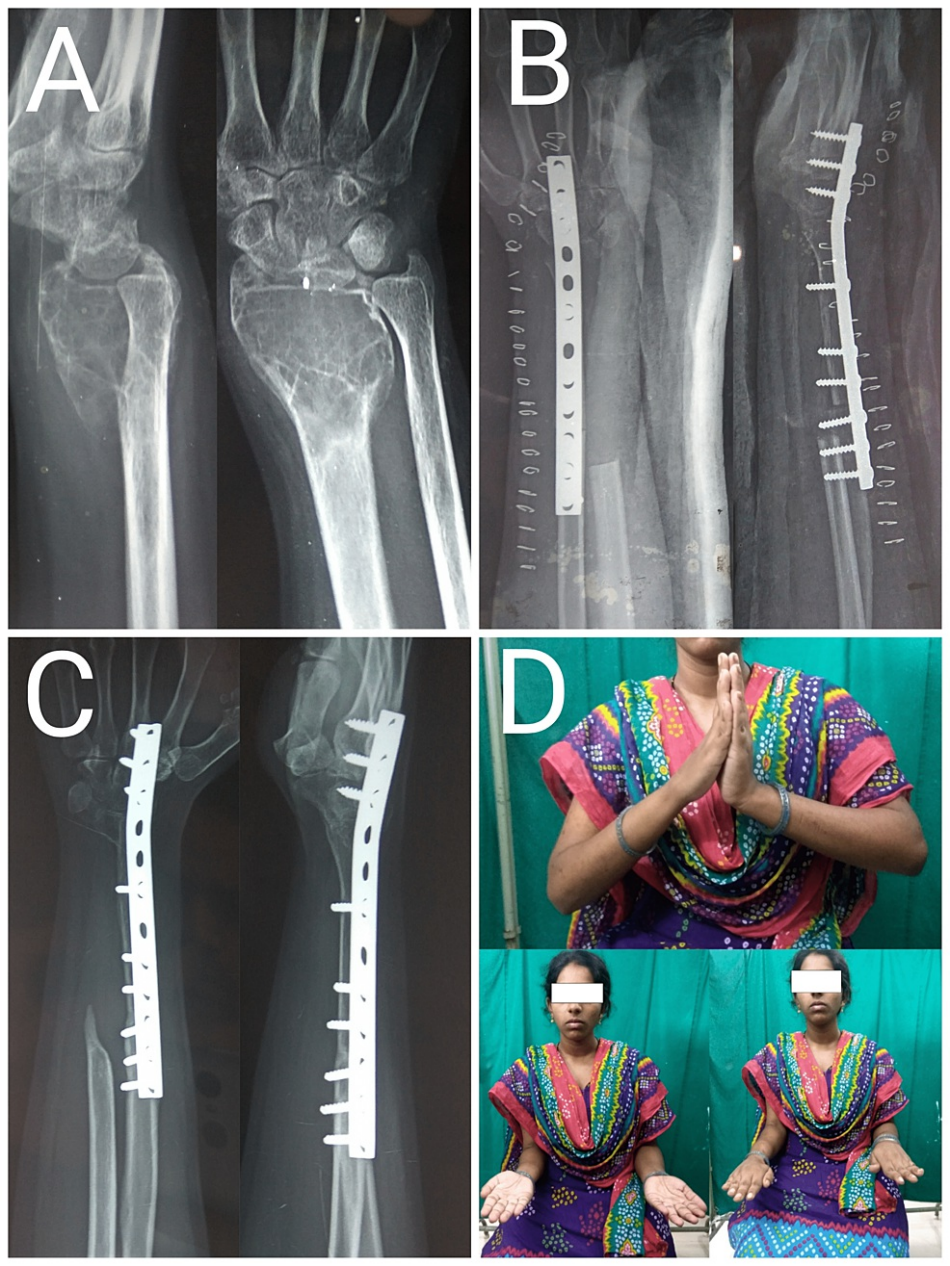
Case images of a 20-year-old female patient with Campanacci grade III giant cell tumor of right distal radius (A) Preoperative radiograph showing giant cell tumor of radius; (B) Immediate postoperative radiograph showing wide excision and ulnar translocation; (C) One-year post-operative radiograph showing union at ulnoradial and ulnocarpal junction; (D) Good forearm rotations and acceptable wrist and hand function
